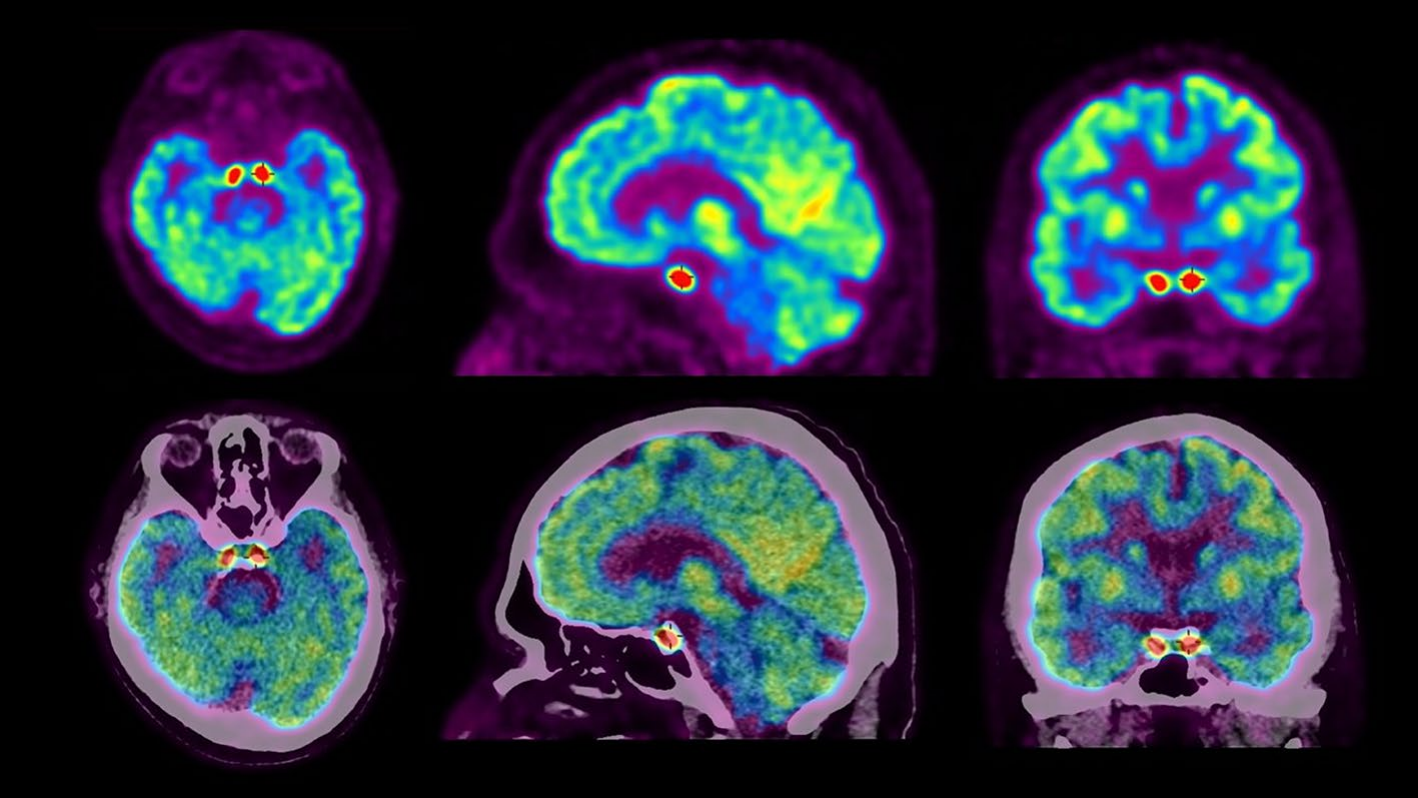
Cerebral ^18^F-FDG PET/CT demonstrating the presence of intense and symmetrical hypermetabolism of the cavernous sinuses
